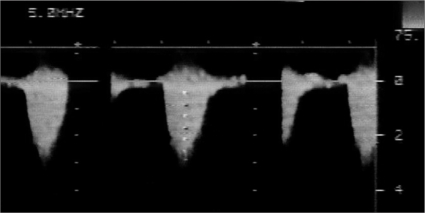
Continuous wave Doppler interrogation across the arch before closure of duct, showing peak systolic gradient of 30 mm Hg, with no diastolic spill
